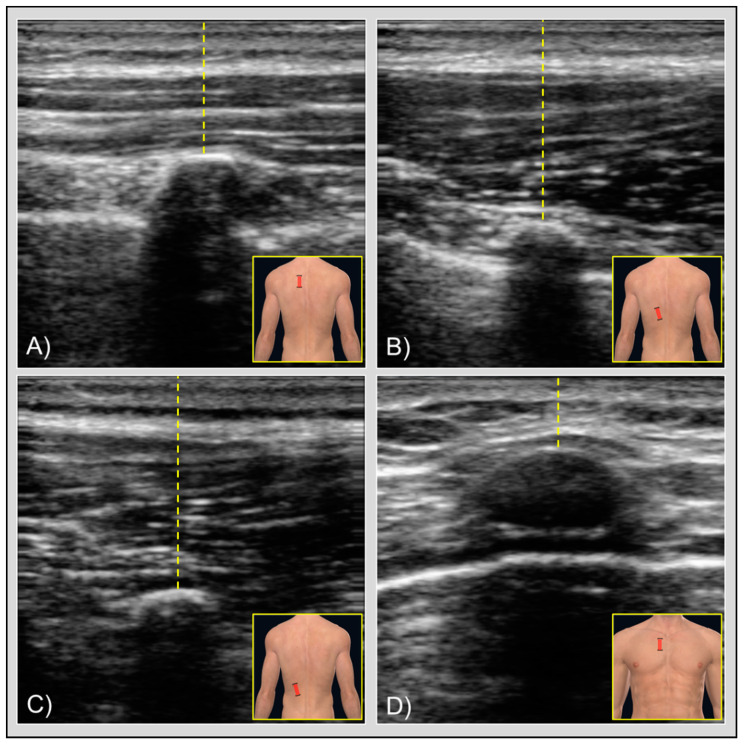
Figures showing the transducer placement (brackets) with ultrasound images and skin-to-rib measurement (yellow lines) for (A) rhomboid muscle, (B) lower trapezius muscle, (C) iliocostalis muscle, and (d) pectoralis major muscle. At the center of each image, the curved hyperechoic area represents the apex of the rib. Below the bone is visible the typical hypoechoic signal, also known as acoustic shadowing. Slightly deeper, in quadrants (A,B,D), you can also notice other hyperechoic tissues lateral to the bone, which represent the pleura. In the rhomboid muscle image (A), the different muscle layers are also visible: directly above the skin the middle trapezius muscle, just below it the rhomboid muscle, and around the rib the intercostal muscles. In the pectoralis major muscle image (D), the rib apex is less visible than in the other images due to the costal cartilages.
